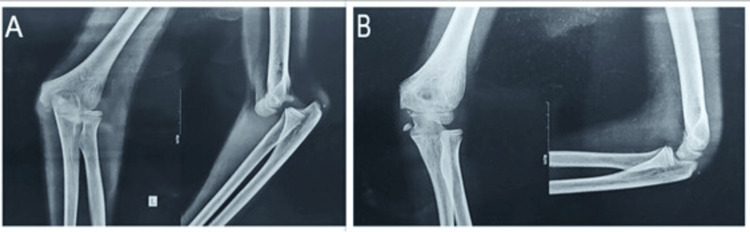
(A) Plain X-ray anteroposterior view and lateral view of the elbow joint after the injury. (B) Plain X-ray anteroposterior view and lateral view after closed reduction
